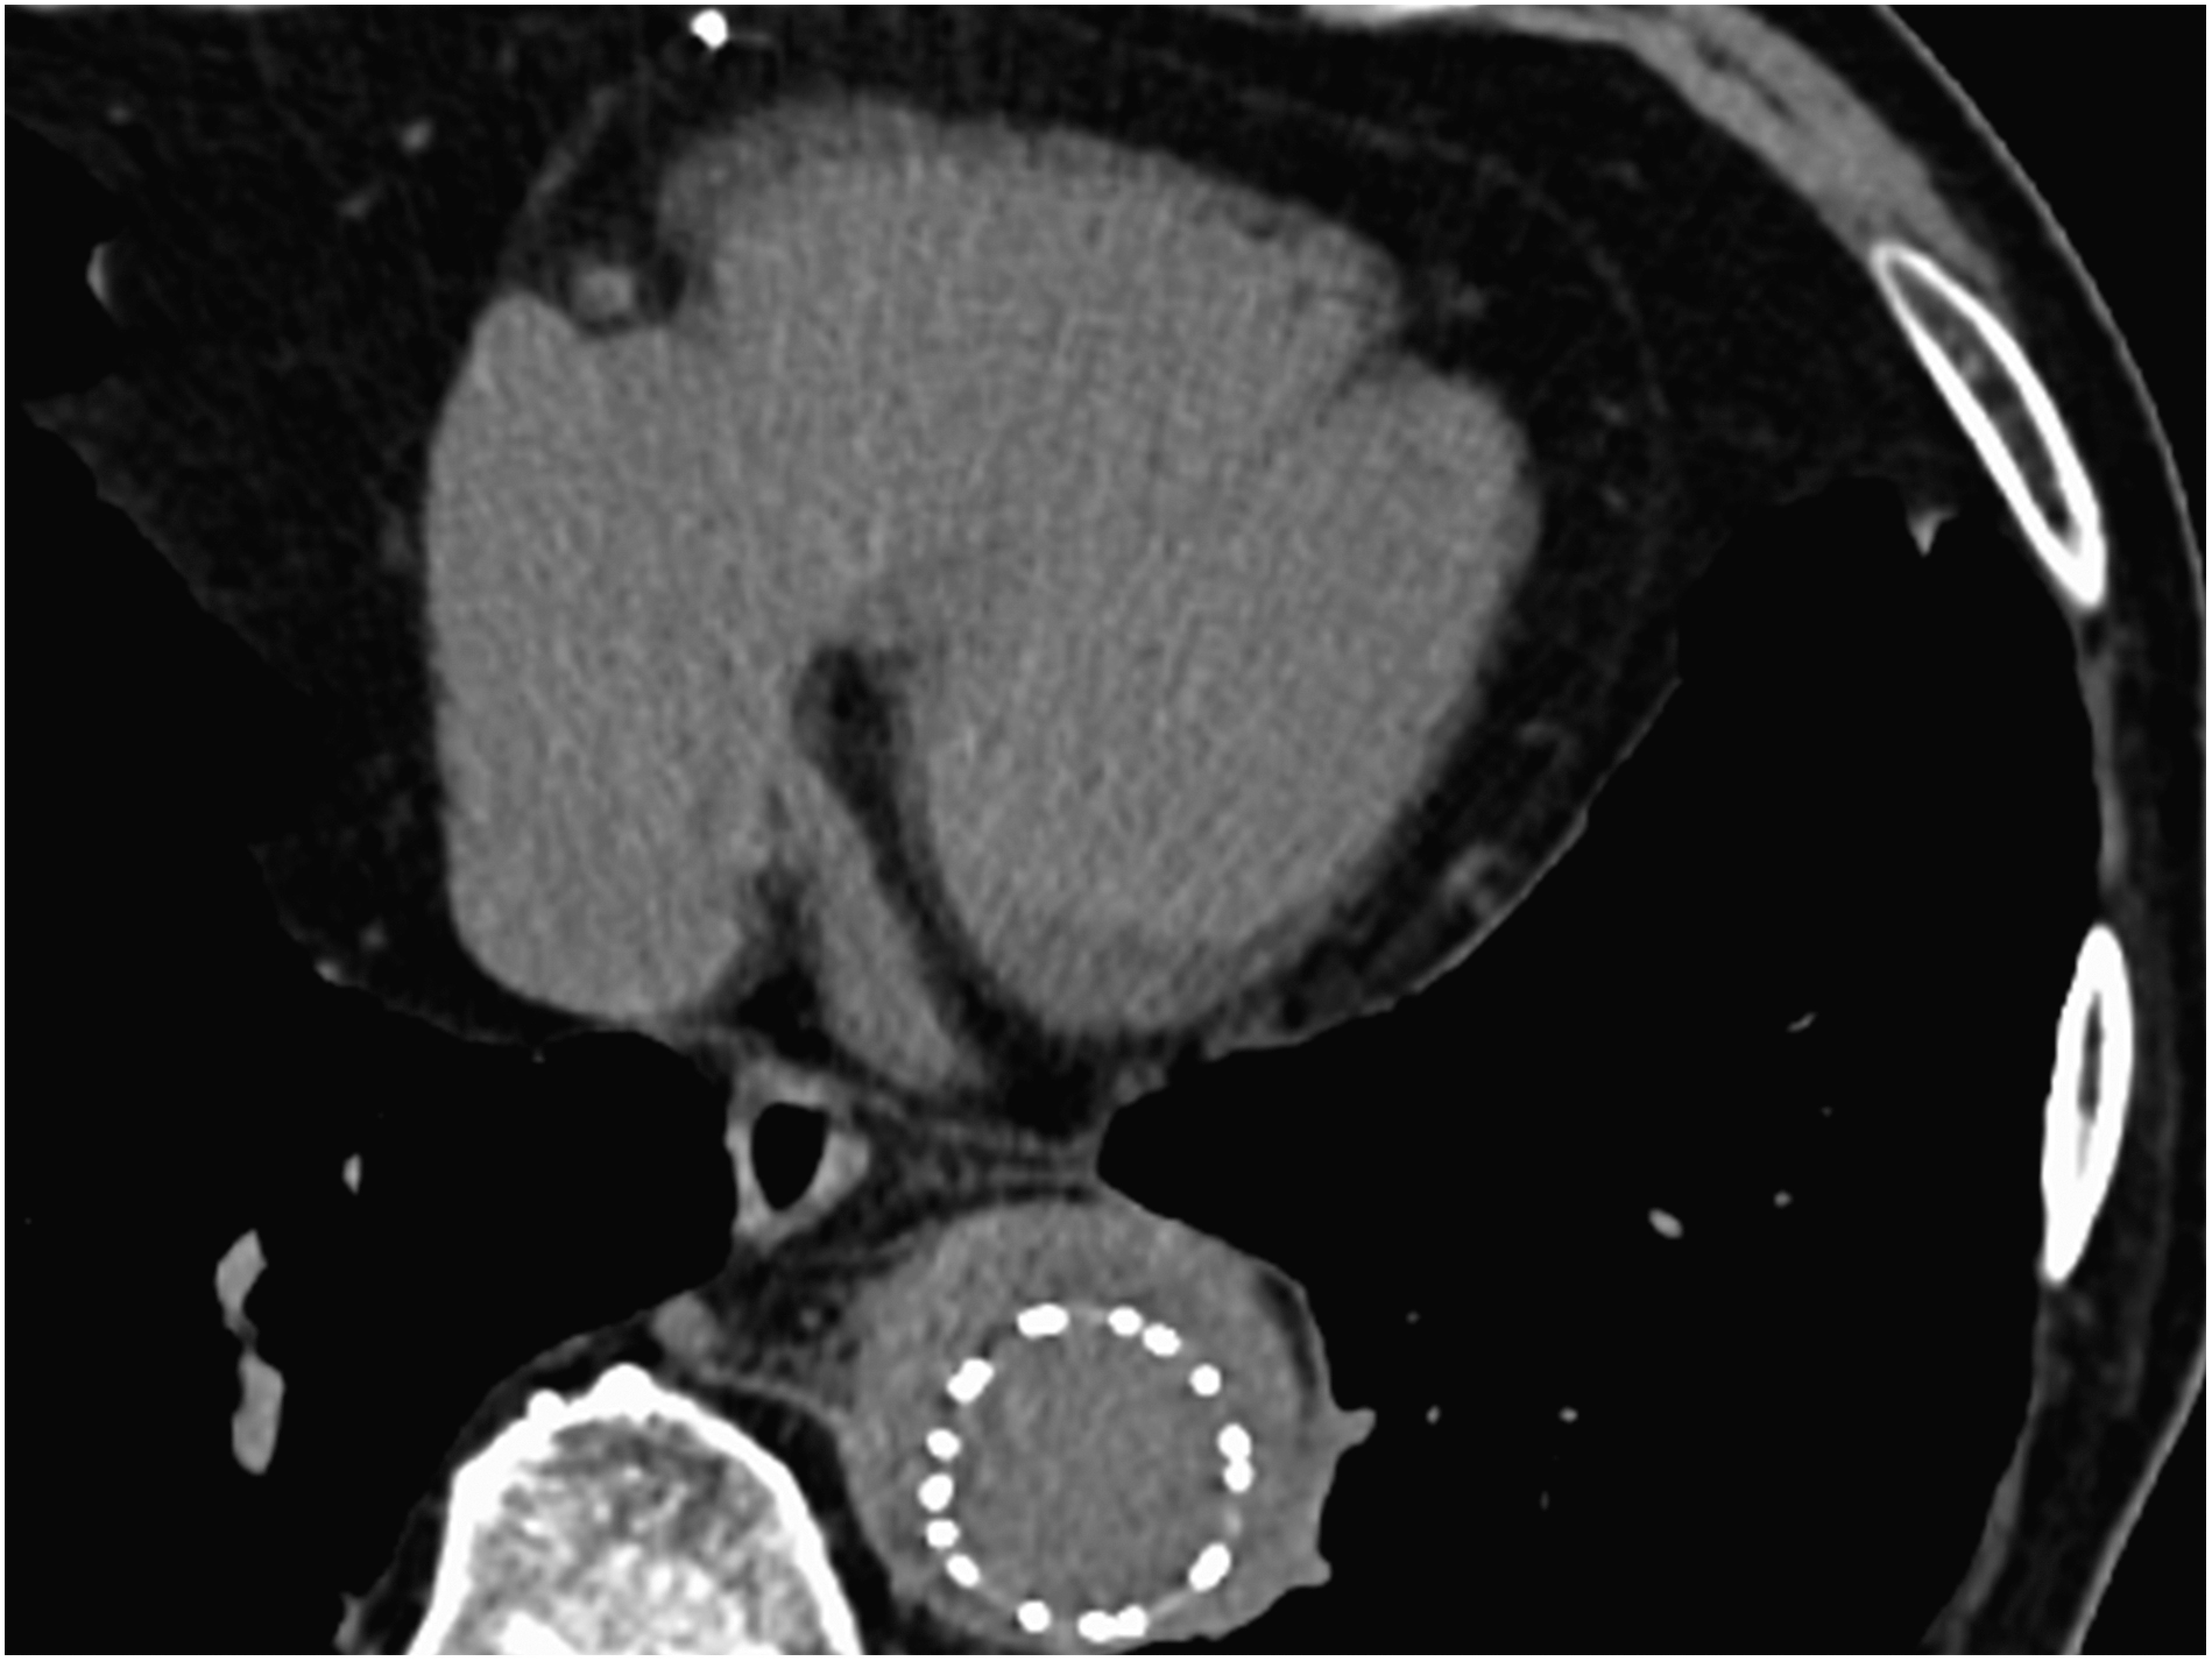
Fig. 3 Non-enhanced computed tomography scan 1 year after surgery was showing significant shrinking of intercostal patch aneurysm without hematoma.
